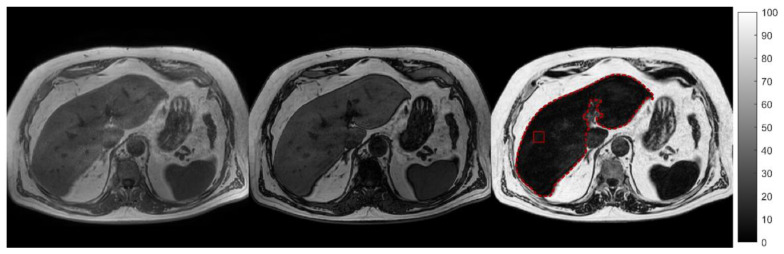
In-phase, opposed phase and MRI-PDFF map segmentation of the entire liver (dotted red line) with the corresponding ROI colocalized with that used for MRS (solid red line).
